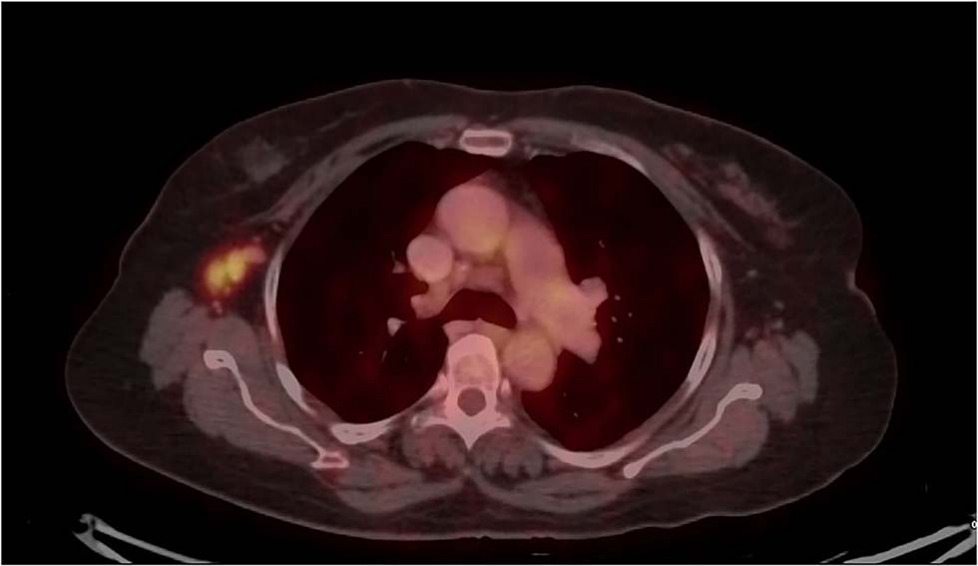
PET-CT axial view of the thorax, indicating a metabolically active lesion in the right axillary region, involving the right axillary lymph nodes.
